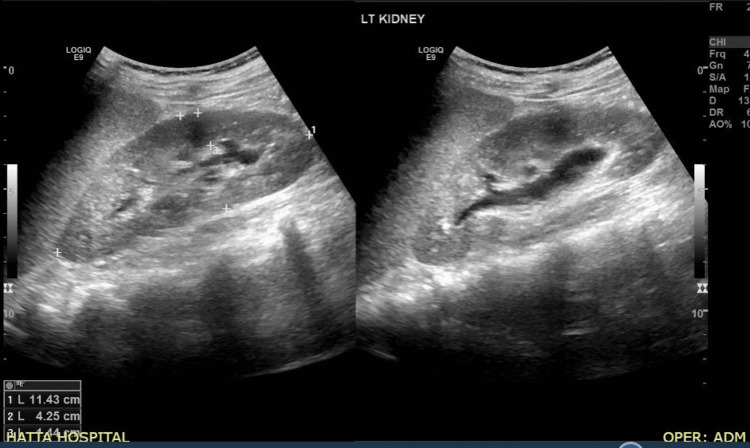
Ultrasonographic Image of the Left Kidney The ultrasound of the left kidney shows mild hydronephrosis, evidenced by slight dilation of the renal pelvis and calyces.
